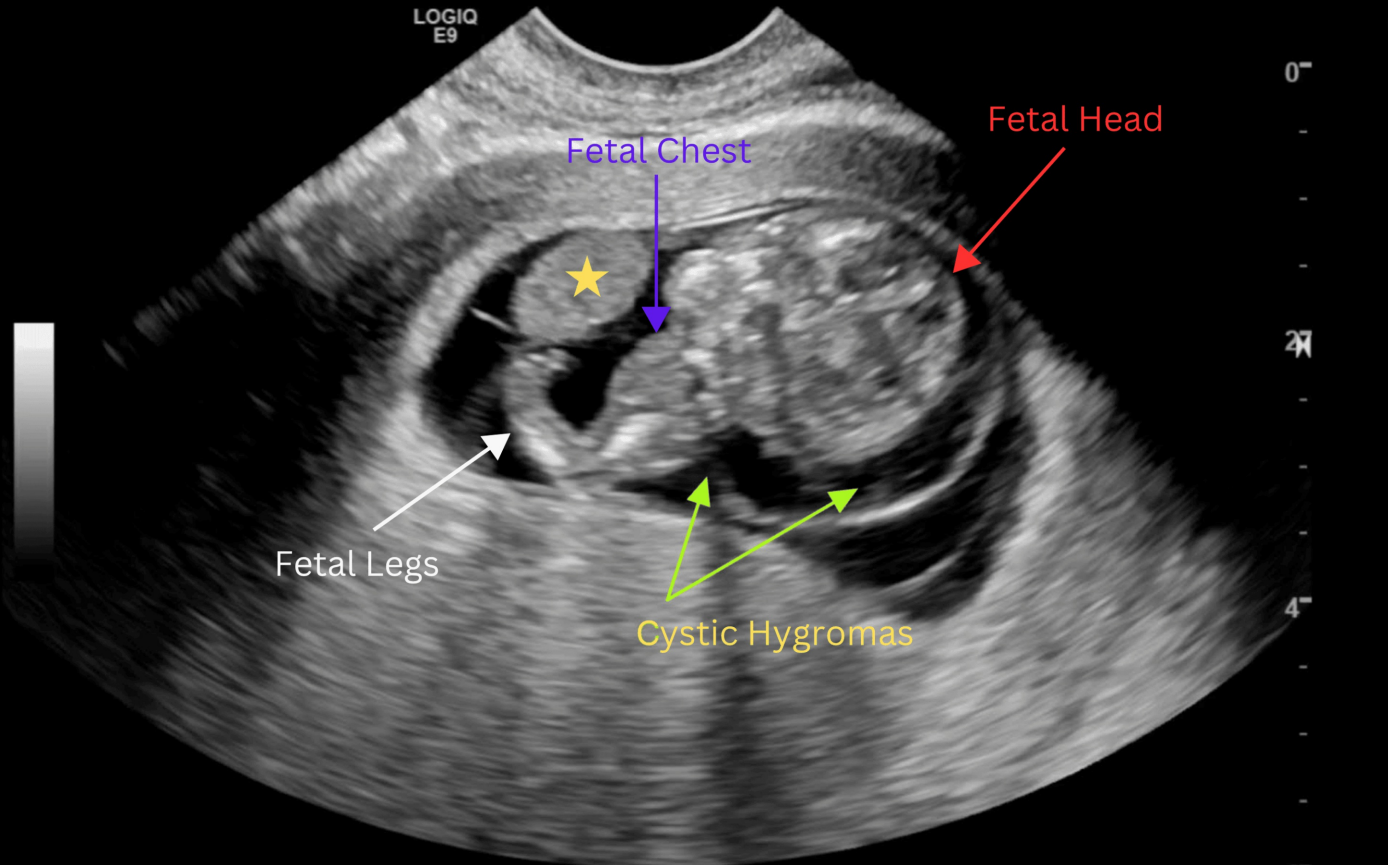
Sagittal ultrasound of the uterus at 11 weeks and four days of gestation, demonstrating findings suggestive of cystic hygroma (yellow arrows) and partial liver herniation (yellow star). Additional landmarks for orientation include the fetal head (red arrow), fetal chest (indigo arrow), and fetal legs (white arrow)
